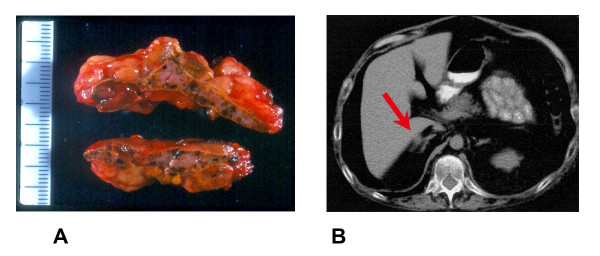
Macroscopic and CT-scan findings in primary pigmented nodular adrenocortical disease (PPNAD) A: Macroscopic appearance of the adrenal gland in PPNAD. The cut surfaces show multiple pigmented micronodules. The periadrenal fat is also visible around the adrenal capsule. B: Adrenal CT-scan in PPNAD. A micronodule is visible on the external part of the left adrenal on the CT-scan shown (see red arrow).
